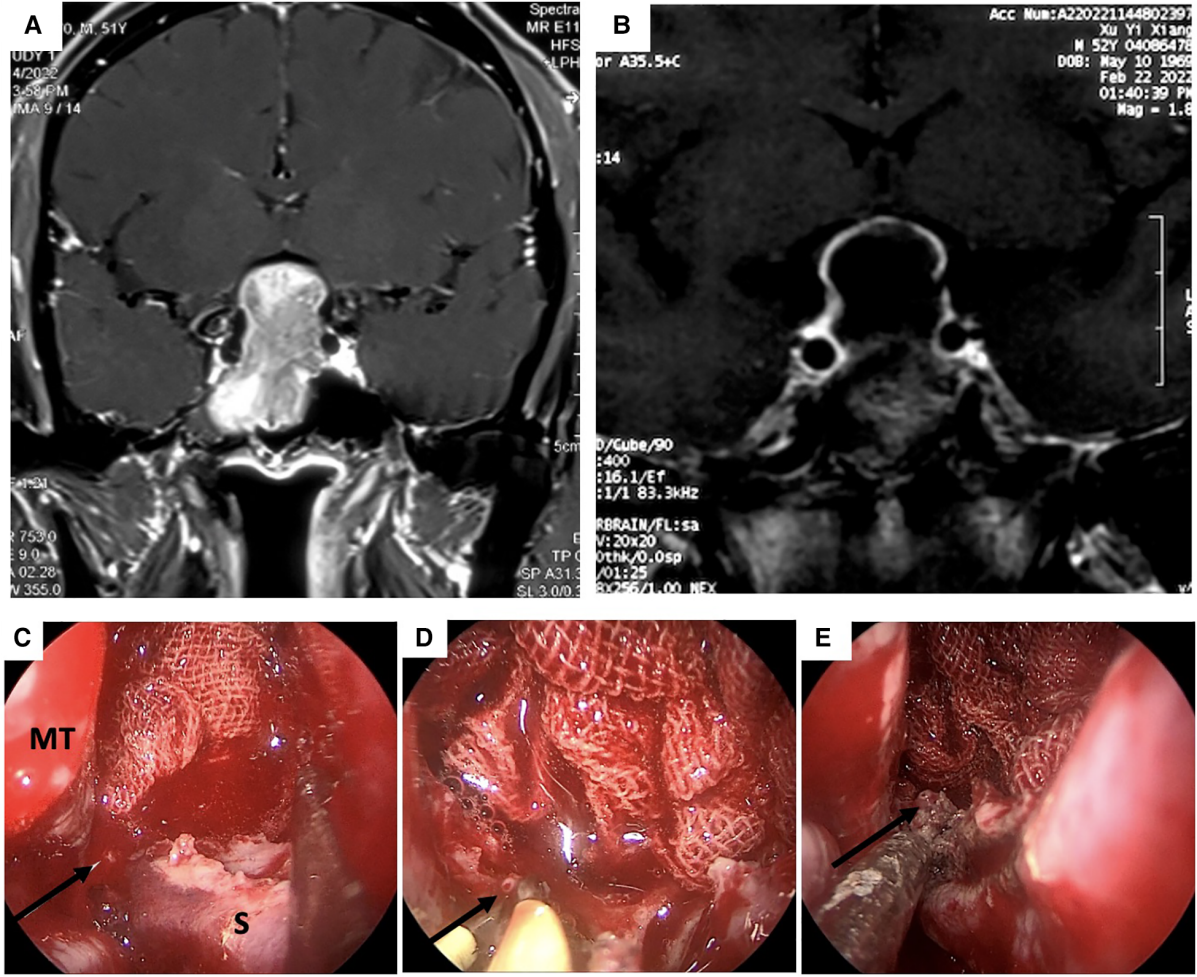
MRI scan and intraoperative view of the epistaxis originated from the right posterior septal artery (PNSA) of case 4. (A and B) pre-and post-operation MRI scan of case 4 (C) The right PNSA was identified as the bleeding source. (D) The PNSA was clamped and cauterized with a cryogenic plasma ablation probe. (E) Hemostasis was successfully achieved. PNSA: posterior nasal septal artery; MT: middle turbinate.
